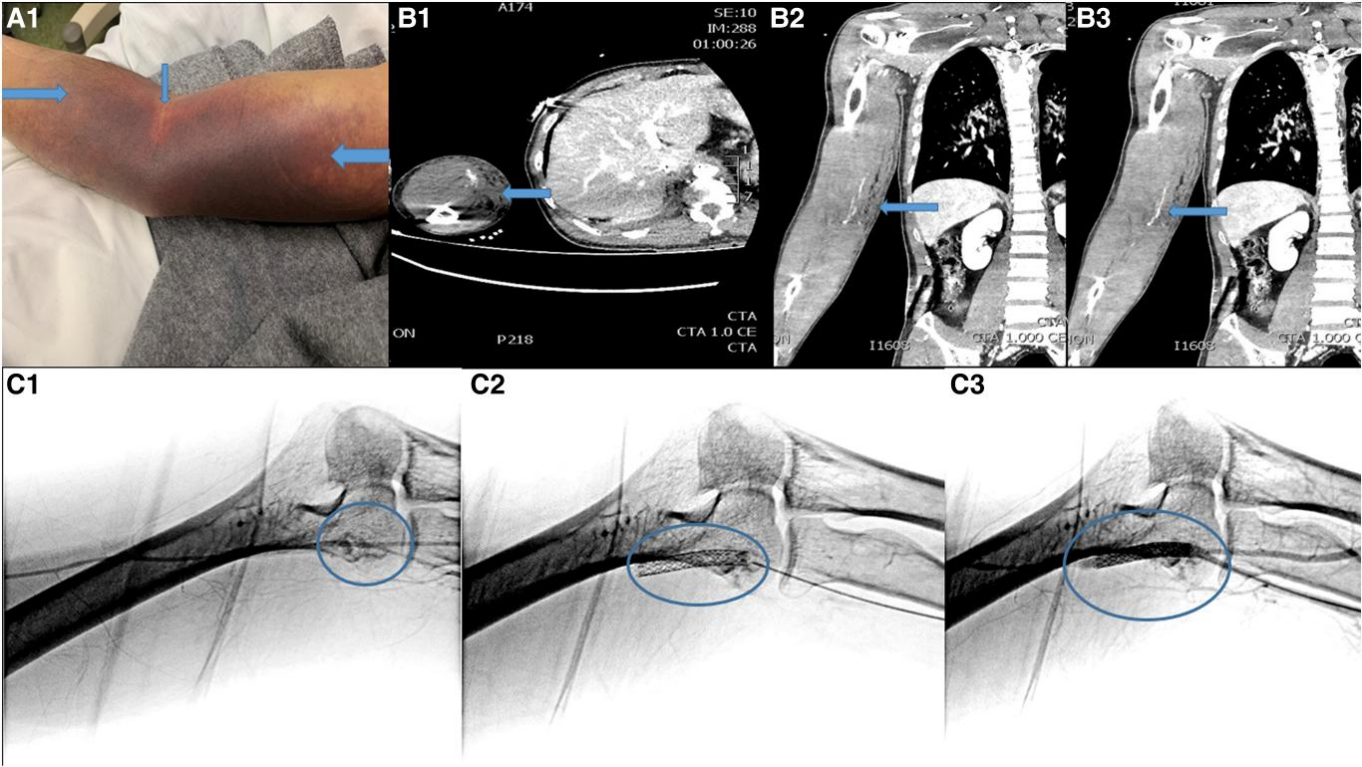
Panel A1*: spontaneous haematoma of right brachial area, *panel B1*: transverse section of computed tomography angiography of right brachial artery showing extravasation and haematoma in right upper limb. *Panels B2-B3*: coronal section of Computed tomography angiography of right brachial artery showing extravasation and haematoma in right upper limb *Panel C1*: angiography of right brachial artery showing extravasation in right brachial artery, *Panel C2*: stent of right brachial artery. *Panel C3*: Angiography of the right brachial artery after deploying the stent, showing no extravasation. (arrows show the haematoma on the right upper limb in brachial area).
